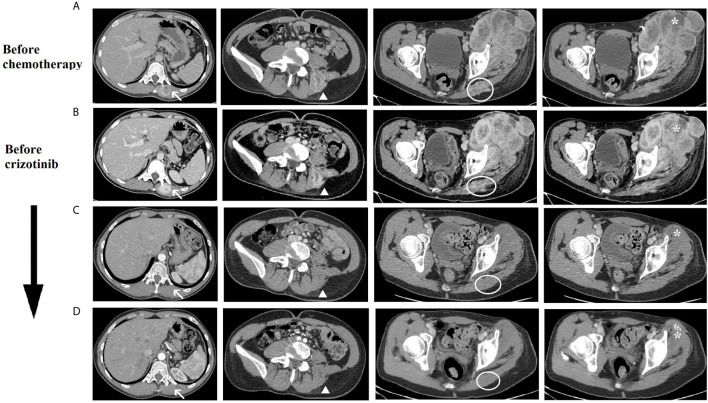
CT scan imaging of the tumoral lesions during patient’s treatment history. Evolution during treatment of the tumoral lesions: lesions of the left dorsal muscles next to D11 vertebral body (arrow) and L5 vertebral body (arrowhead), lesion of the left gluteus (circle) and left thigh mass (asterisk). (A) before chemotherapy (March 2019), (B) after second cycle of chemotherapy and before crizotinib (May 2019), (C) after 4 months of crizotinib (September 2019), (D) after 7 months of crizotinib (December 2019).
